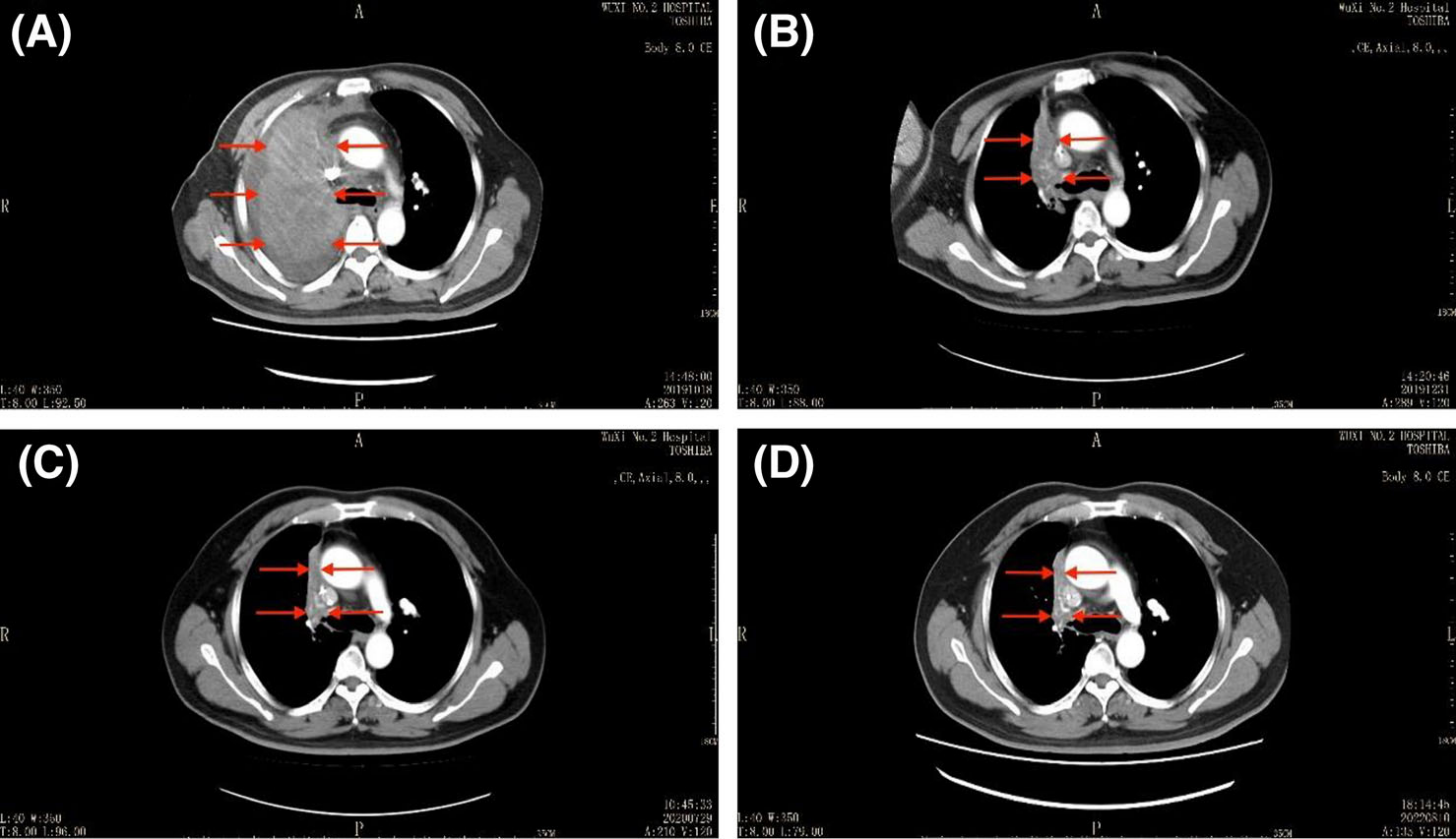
Contrast‐enhanced computed tomography (CT) scans of the histiocytic sarcoma patient before and after immunotherapy, showing the lesions were significantly reduced and remained stable for nearly 3 years. (A) 18 October 2019, 141 × 77 mm; (B) 31 December 2019, 90 × 24 mm; (C) 29 July 2020, 72 × 12 mm; (D) 10 August 2022, 70 × 8 mm.
